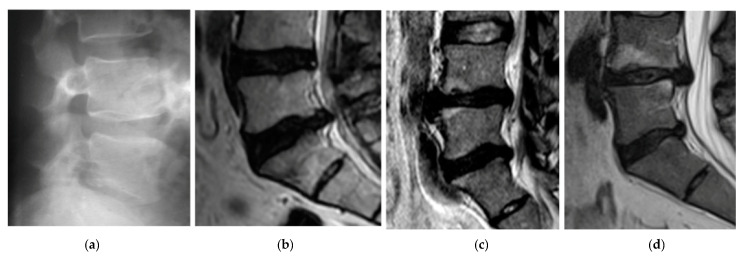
Sagittal plane magnetic resonance imaging (MRI) cases: A—case (a) B—case (b) C—case (c) D—case (before surgery) (d).
